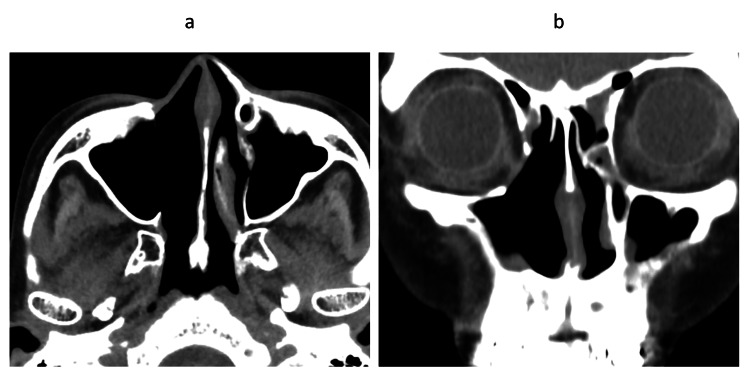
Postoperative follow-up CT images three years after surgery showing no evidence of recurrence (a) Axial view and (b) coronal view. No recurrence is observed in either image. CT: computed tomography
